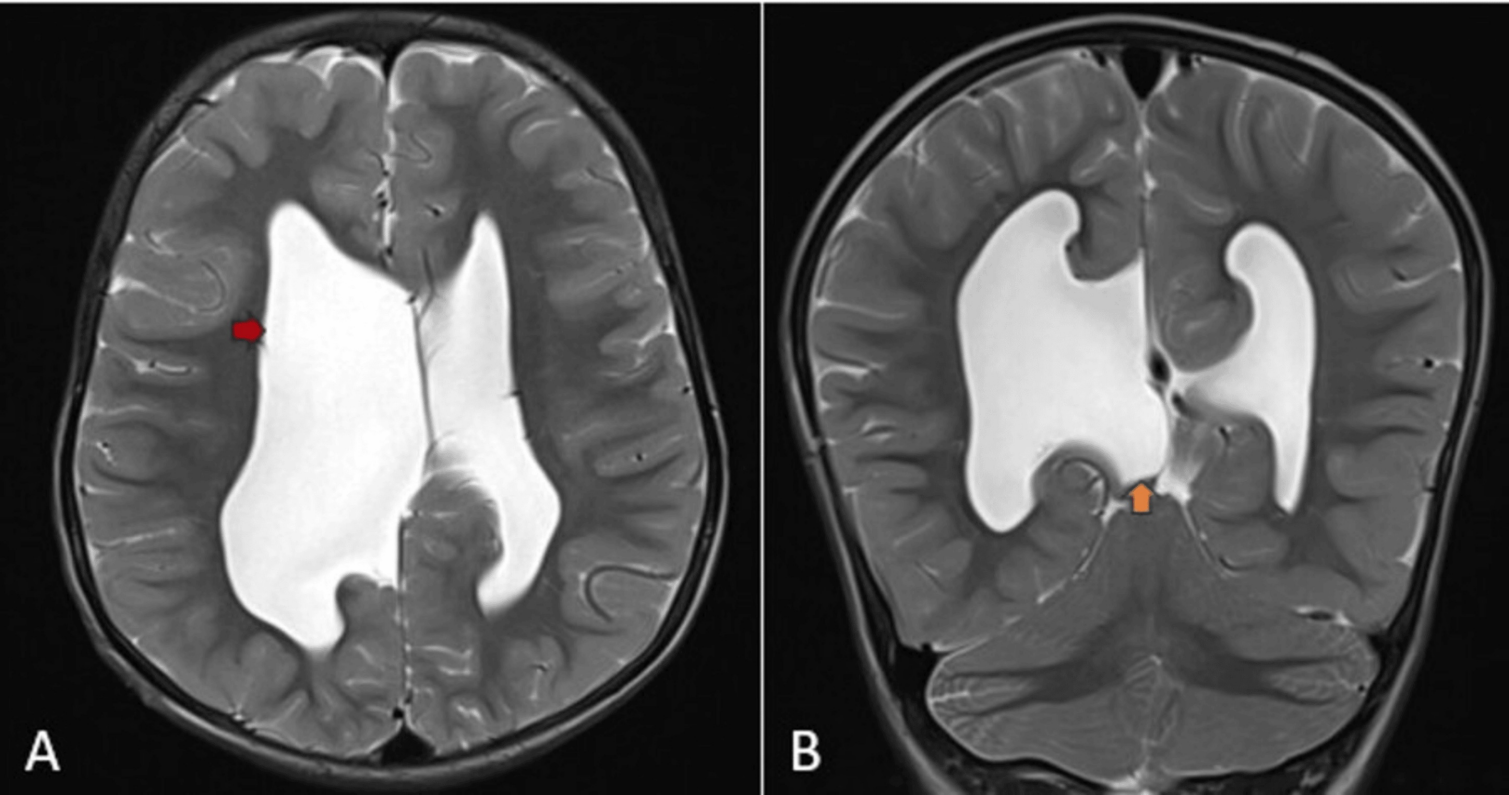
MRI T2WI images Image A (axial) shows asymmetrical dilatation of the lateral ventricles (red arrow). Image B (coronal) demonstrates a dorsal interhemispheric cyst (yellow arrow) MRI: magnetic resonance imaging; T2WI: T2-weighted imaging
